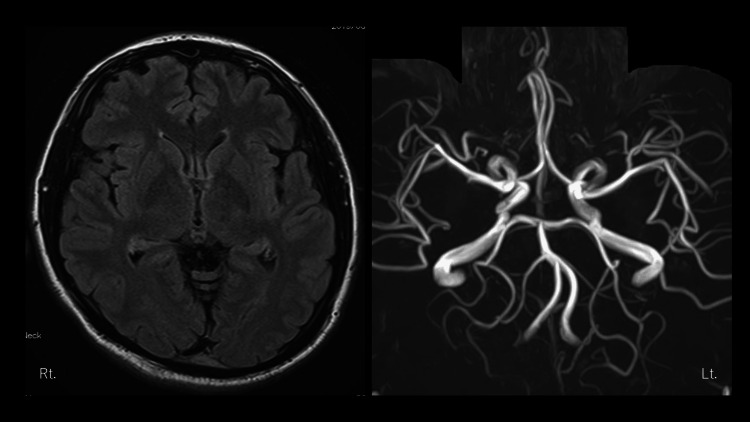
Head-plane magnetic resonance imaging and magnetic resonance angiography at initial visit. There were no apparent organic diseases.
